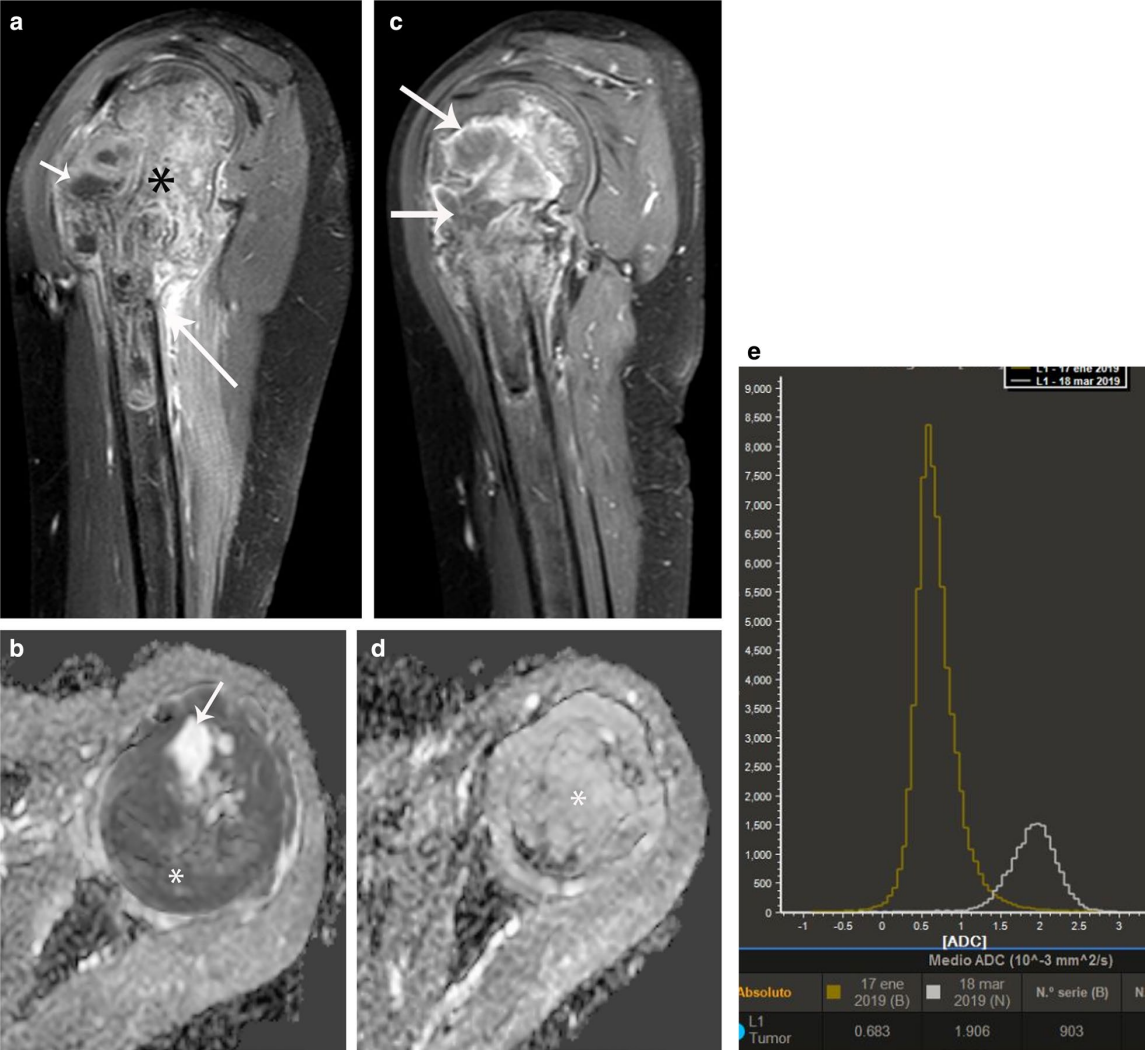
Ewing sarcoma involving the proximal left humerus in a 12-year-old boy. a Sagittal contrast-enhanced fat-suppressed T1-weighted MR image shows a heterogeneous mass centered in the proximal humeral metaphysis (*) that extends to the epiphysis and proximal diaphysis and contains foci of internal necrosis (short arrow). Note severe varus angulation of the humerus due to previous fracture (long arrow). b Axial ADC MR image shows restricted diffusion of the cellular areas (*) with no restriction of the necrotic foci (arrow). c Sagittal contrast-enhanced fat-suppressed T1-weighted MR image after treatment shows decrease in volume of the mass with increase of necrotic areas (arrows). d Axial ADC MR image after treatment shows no restricted diffusion (*). e Volumetry of ADC maps performed before treatment shows a high and sharp ADC histogram of 0.7 mm^2/^s, while the histogram after treatment is short and wide and shifted to the right, with a value of 1.9 mm^2/^s. The conventional MRI sequences together with DWI indicate good treatment response
